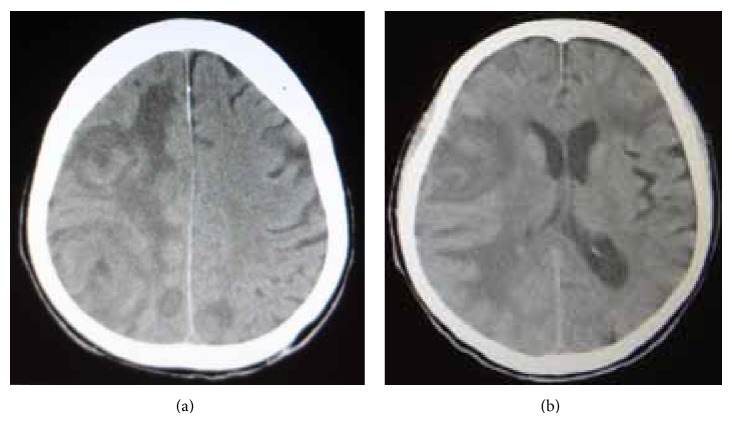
Following surgical operation and radiation therapy, computed tomography (CT) showed a large recurrent tumor with perifocal edema in the right frontal lobe (a) (b).
